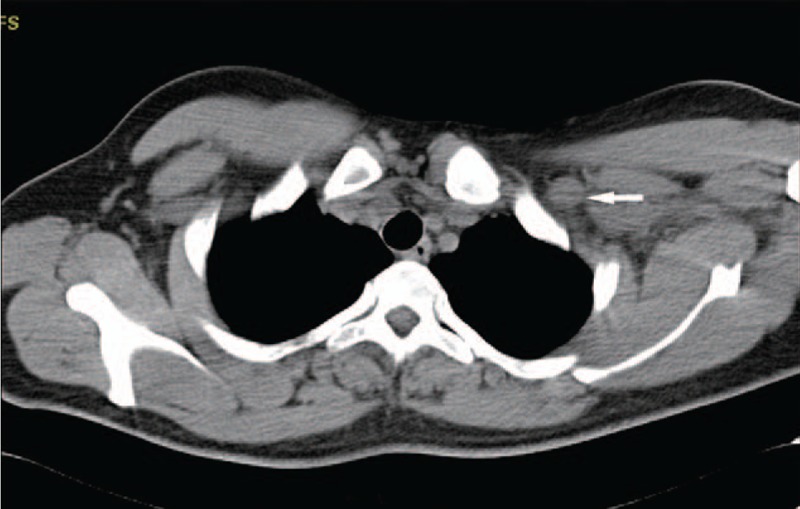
Chest CT showed an enlarged lymph node in the infraclavicular region (arrow) after the first surgery. CT = computed tomography.
